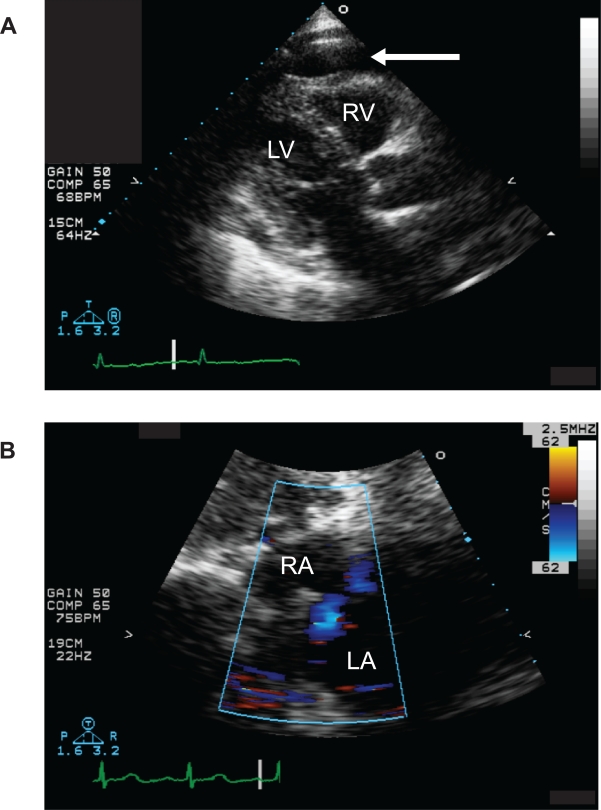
Panel A) TTE, parasternal long-axis view: a moderate, circumferential pericardial effusion is visible, with no evidence of pericardial mass or significant fibrin deposition (arrow). The silhouette of the right ventricular outflow tract, marked RV, reveals inversion of the free wall, indicating an increase in pericardial pressure. The left ventricle (marked LV) is also clearly visible in this view. Panel B) TTE, subcostal view with Doppler: the right atrium is marked RA and the left atrium is marked LA. The Doppler scan reveals a jet moving away (colored blue) from the probe, from the right to the left atrium.
